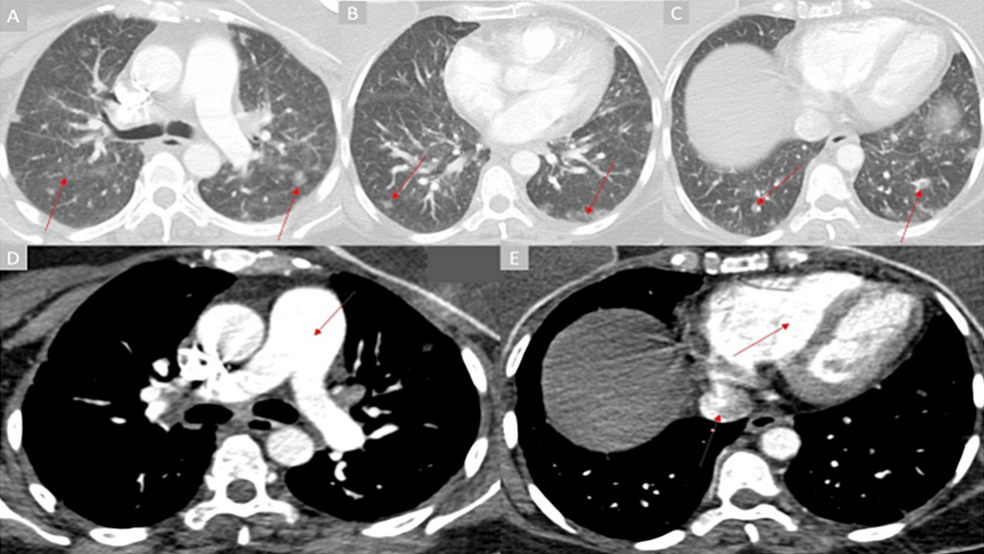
Chest CT Panels A through C: axial lung window views demonstrate the perivascular ground glass opacities and ill-defined centrilobular nodules (red arrows). Panel D: axial mediastinal window view shows an enlarged main pulmonary artery (red arrow). Panel E: axial mediastinal window view shows an enlarged right ventricle and reflux of contrast into the inferior vena cava (red arrows).
